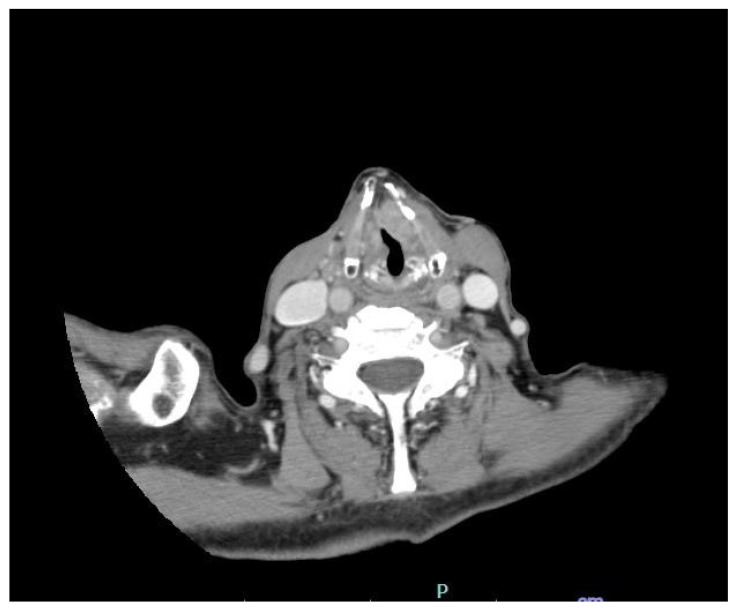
CT Scan demonstrated irregularity of the anterior glottic contour with involvement of the anterior commissure and contrast enhancement of the mucosa and submucosa, along with obliteration of the paralaryngeal fat planes.
